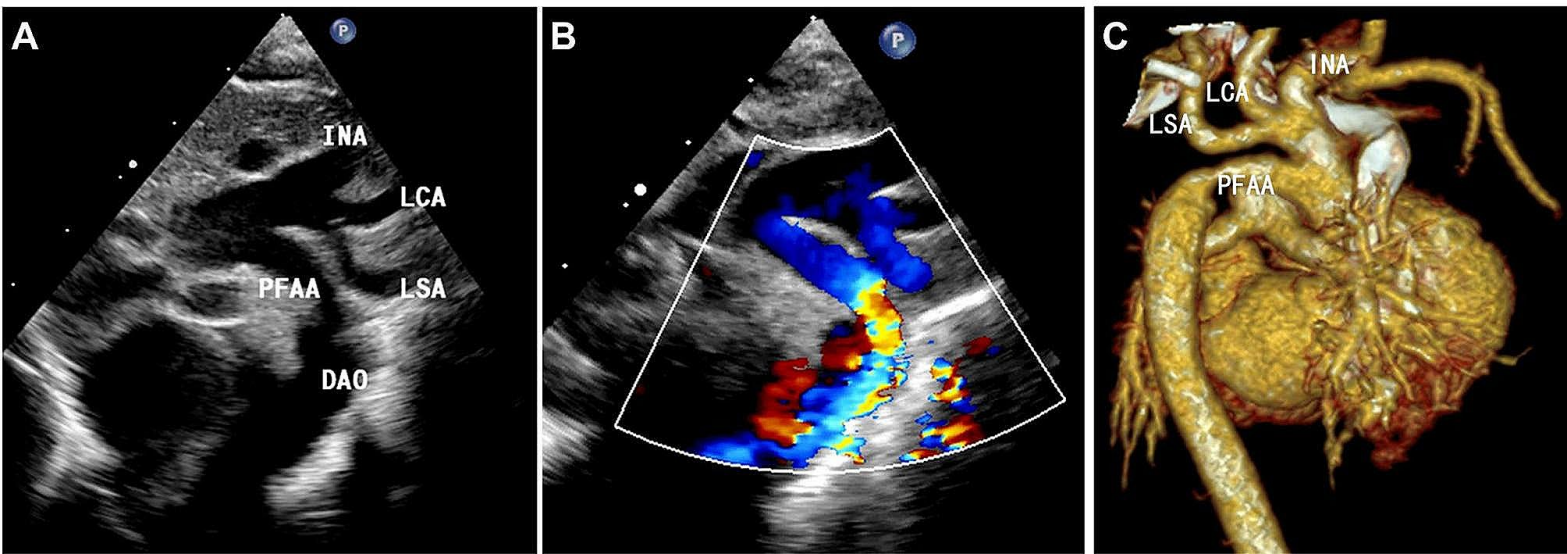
Case 4, Weinberg type B PFAA combined with aortic coarctation. (A & B): Echocardiography showing the interrupted fourth aortic arch (superior) and fifth aortic arch (inferior) connecting to the descending aorta. (C): Confirmation via computed tomography. DAO, descending aorta; INA, innominate artery; LCA: left common carotid artery; PFAA, persistent fifth aortic arch
